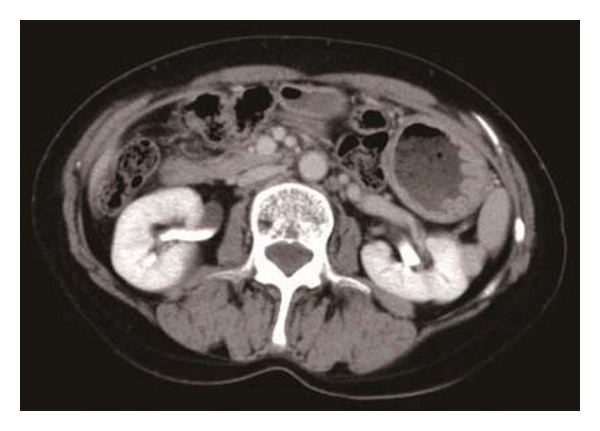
Contrast-enhanced computed tomography showing a homogenously enhanced lenticular tumor from the lateral aspect of the middle pole of the left kidney.
